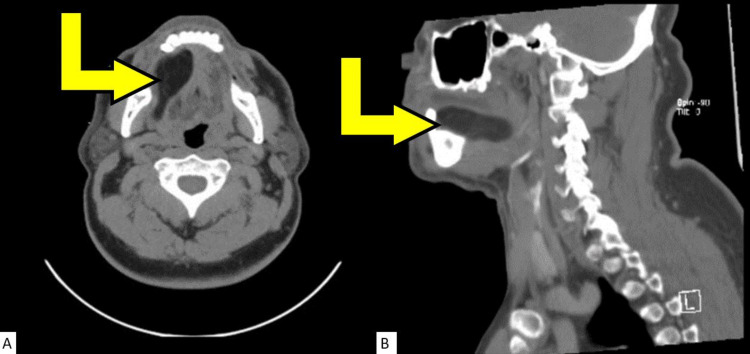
Computed tomography (CT) scans of the patient's head and neck without contrast. The yellow arrows in both images are pointing at the mass in the patient's sublingual area. The low attenuation and smooth borders of the lesion are indicative of a fatty mass. (A) Axial CT scan. (B) Sagittal CT scan.
